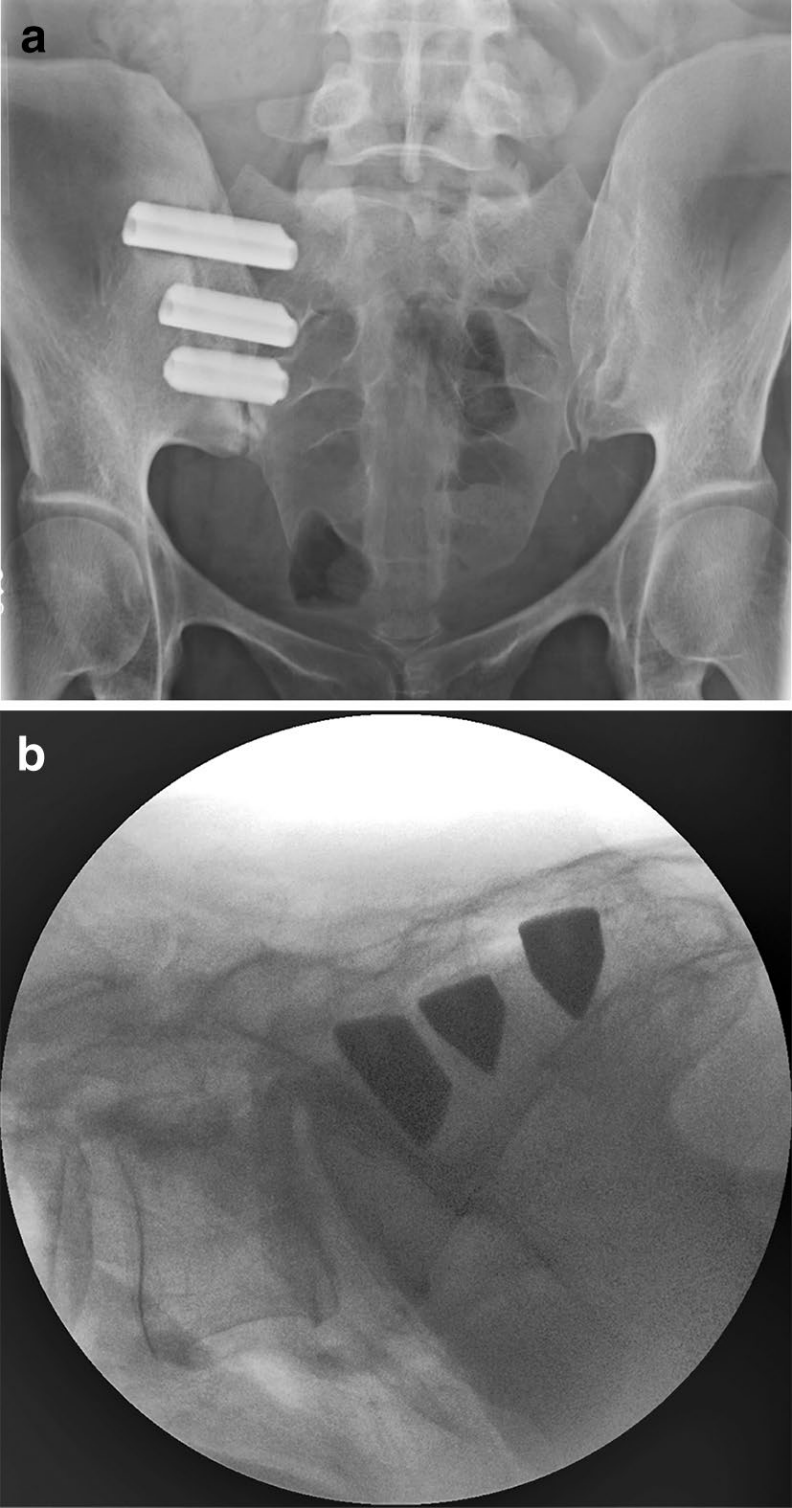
a Pelvic AP and b lateral plain film radiographs showing three implants placed across the SI joint
